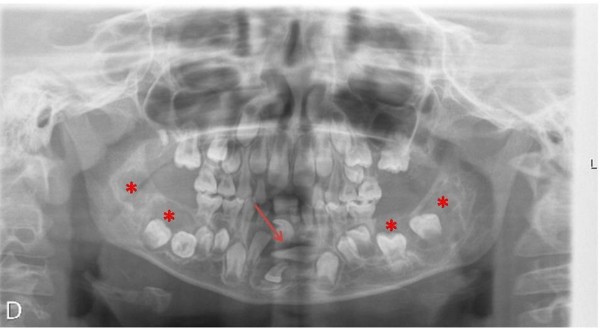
Panoramic radiography of the nephew, affected. Arrow: follicular cyst in piece 43. Asterisks: odontogenic cysts in posterior mandibular regions and right mandibular area.
